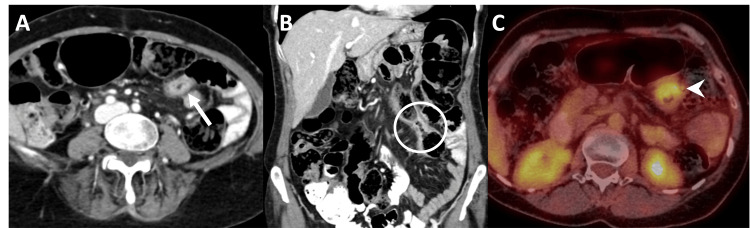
Breast cancer metastasis to the colon. (A) Axial contrast-enhanced CT showing infiltrative mural thickening and hyperenhancement of the transverse colon (arrow). (B) Coronal contrast-enhanced CT demonstrating the same lesion (circle). (C) Axial 18F-FDG PET-CT showing increased FDG uptake (arrowhead) corresponding to the colonic wall thickening and hyperenhancement. ^18^F-FDG PET-CT: Fluorine-18 fluorodeoxyglucose positron emission tomography/computed tomography; CT: Computed tomography; FDG: Fluorodeoxyglucose
